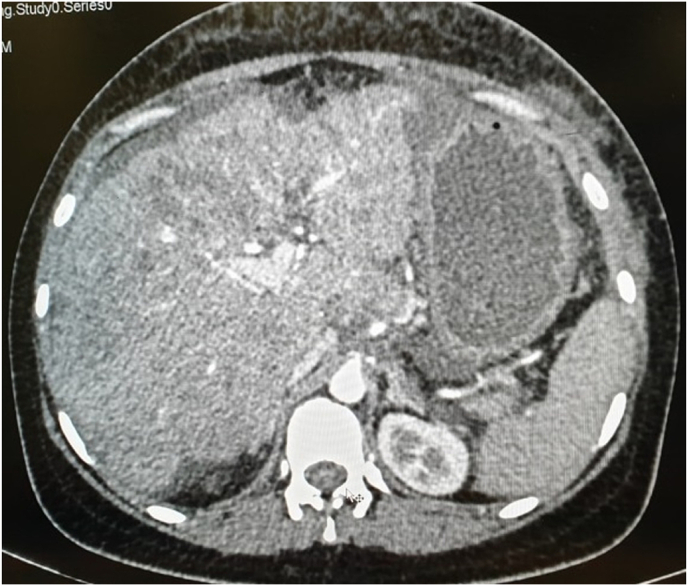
CT arterial phase (Liver). Legend: An irregular liver with several hypoechoic lesions with slight enhancement and ascites.
